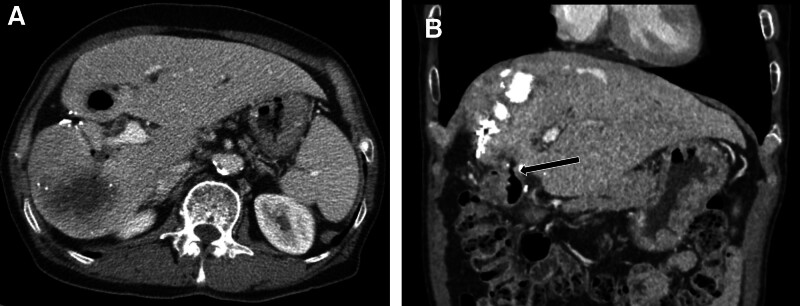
Two-month postoperative CT scans. (A) A 2 cm–sized oval air density lesion instead of a lipiodolized tumor in S4b. (B) Communication between the lesion and the duodenal lumen (arrow). CT = computed tomography.
